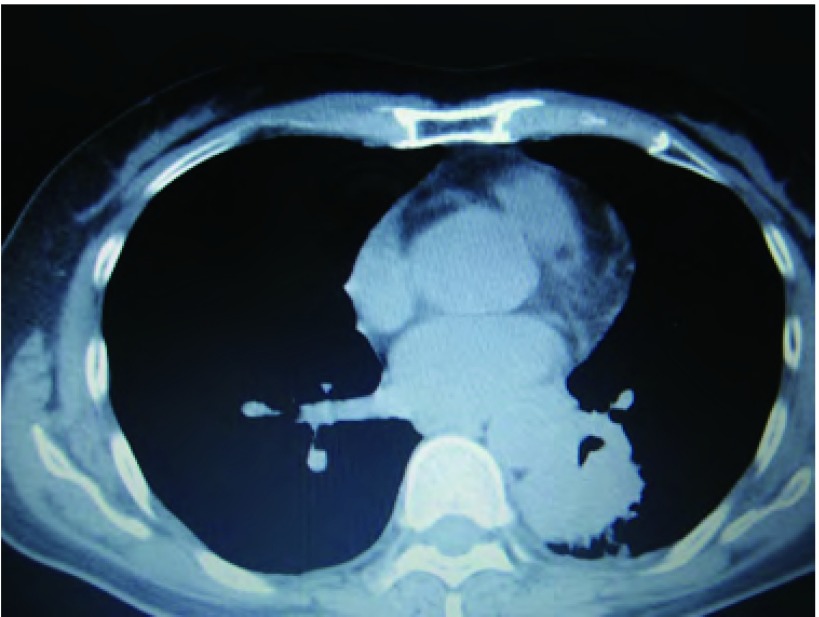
患者临床影像学特征。胸CT示左下肺中央型肺癌。 Clinical radiologic features of the patient. The CT of chest showing left lower lung central type lung cancer.
